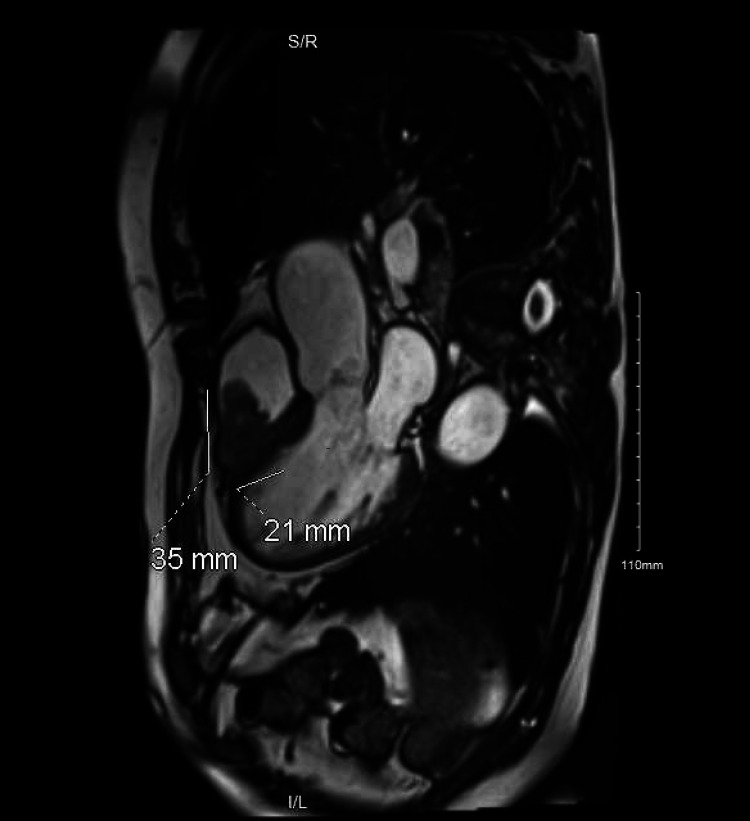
Cardiac MRI (2) Sagittal view from cardiac MRI showing RV mass with measurements. RV: right ventricle
